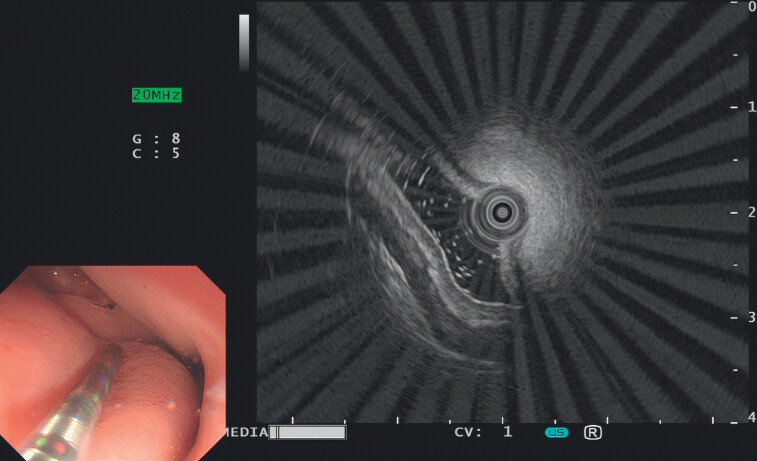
Endoscopic ultrasound demonstrating a well-defined homogeneous
